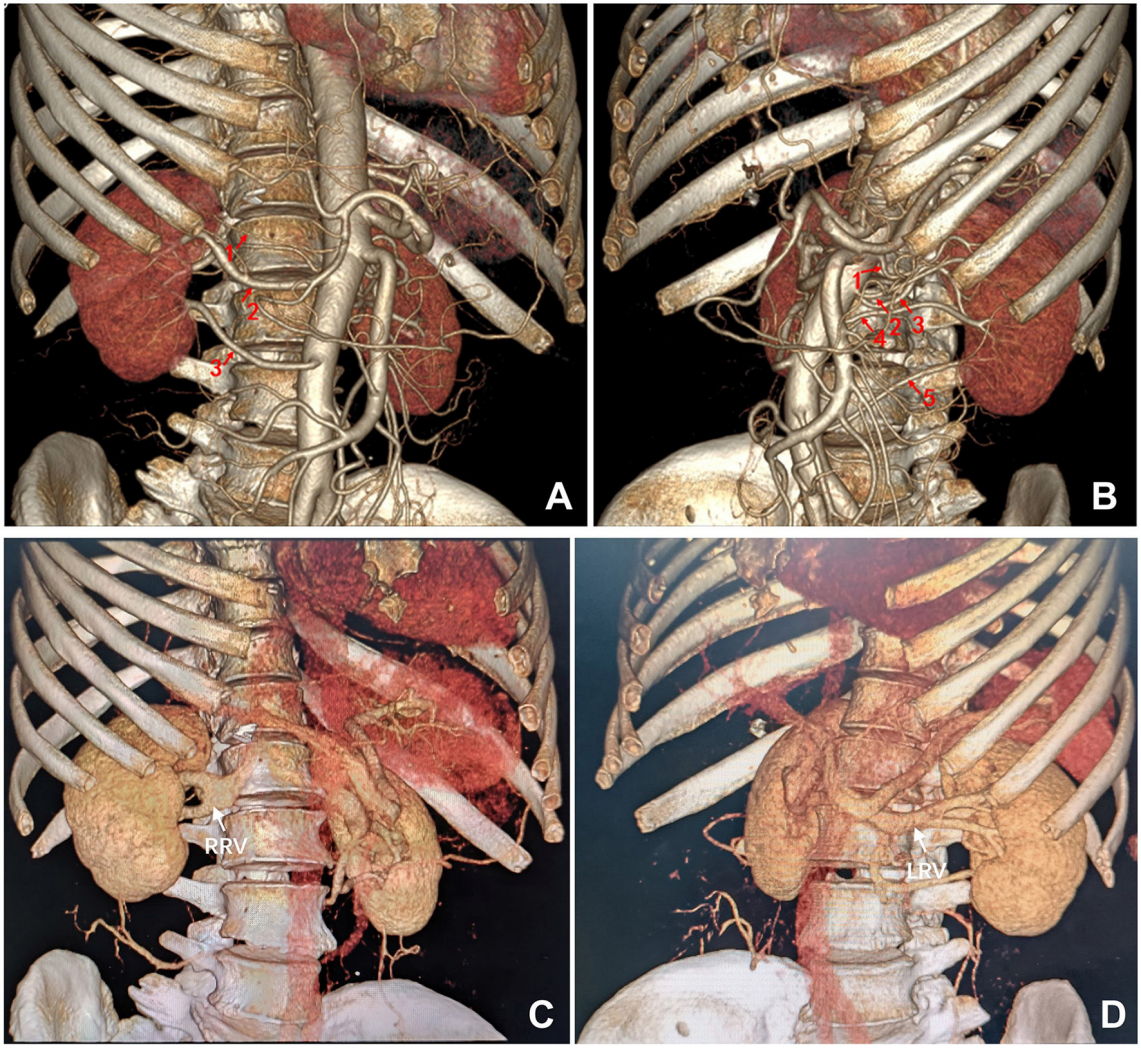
Preoperative computed tomography angiography of the donor. (A) Three renal arteries supplying the right kidney. (B) Five renal arteries supplying the left kidney. (C) Two right renal veins forming a common trunk. (D) Two left renal veins forming a common trunk. LRV, left renal vein; RRV, right renal vein.
